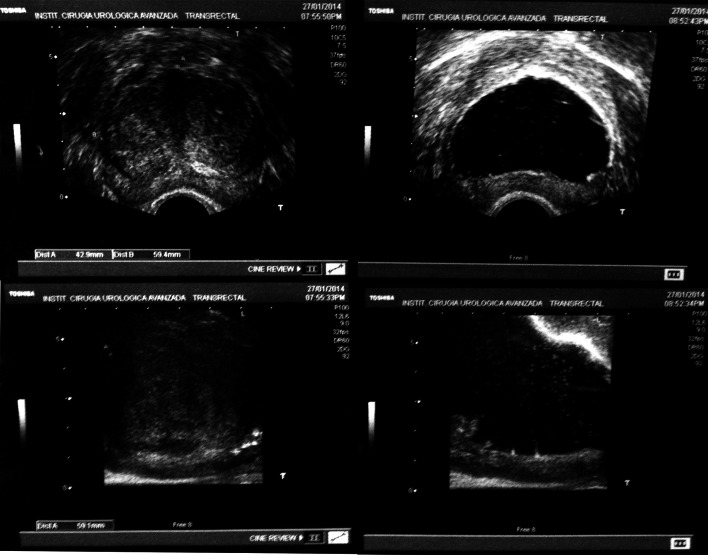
Pre- and postoperative transrectal ultrasound of the prostate showing the excellent removal of the adenoma
